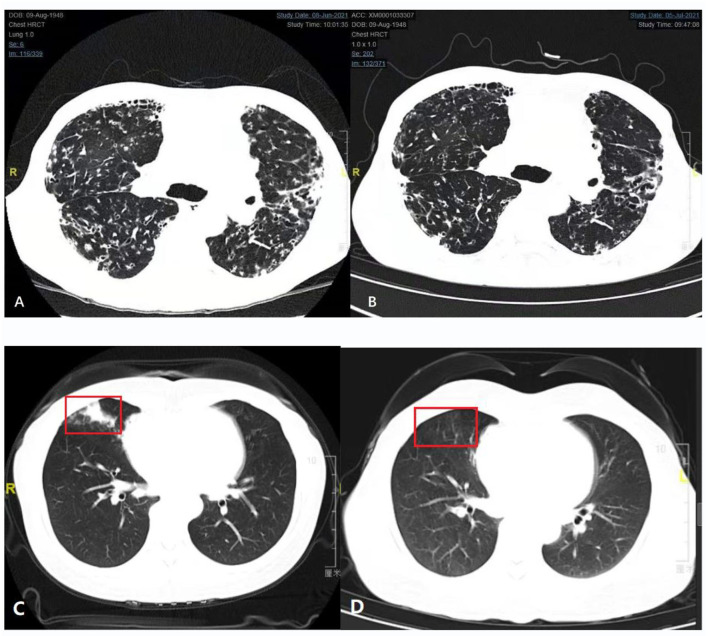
Comparison of chest computed tomography (CT) before and after treatment (Case 16X and Case 17X). (A,B) Comparison of chest computed tomography (CT) before and after 4 weeks of antibiotic treatment (Case 16X). (A) Plain chest CT scan before antibiotic therapy. (B) Plain chest CT scan after 1 month of antibiotic therapy. (C,D) Comparison of chest CT before and after 3 months of antibiotic treatment (Case 17X). (C) Plain chest CT scan before antibiotic therapy. The red box is a typical *Nocardia* infection site. (D) Plain chest CT scan after 3 months of antibiotic therapy. Inflammation of the red box had subsided.
